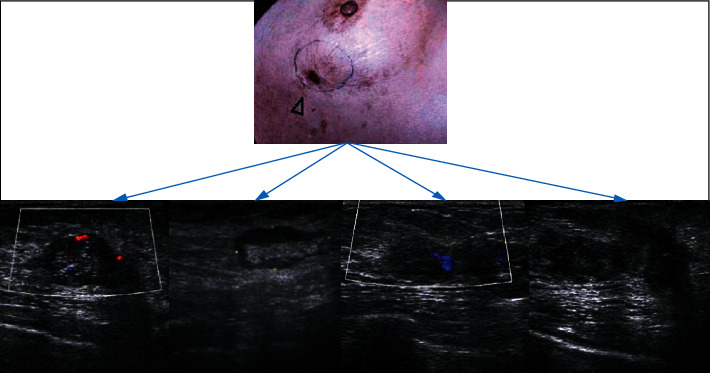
The ultrasound images of a 46-year-old female patient with breast cancer. The patient went to hospital for examination due to a lump in the left breast with obvious pain.
